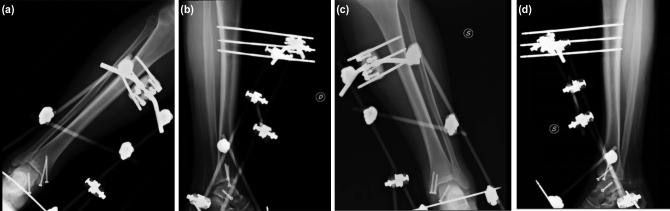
(a), (b), (c) and (d) Post-operative radiograph, show the anatomical reduction of both ankles.
